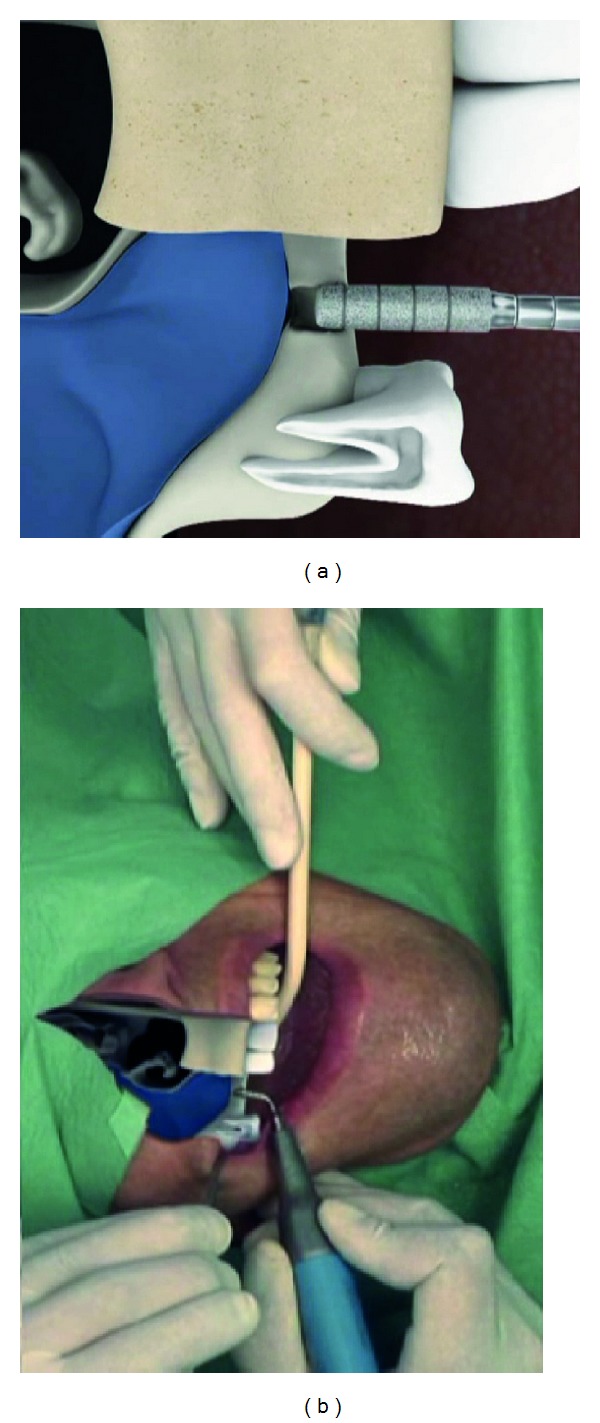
Intralift: preparation of the receptacle for the hydrodynamic cavitational ultrasound applicator with the diamond-coated tip TKW4 for Piezotome (preparation of a ventile seat).
